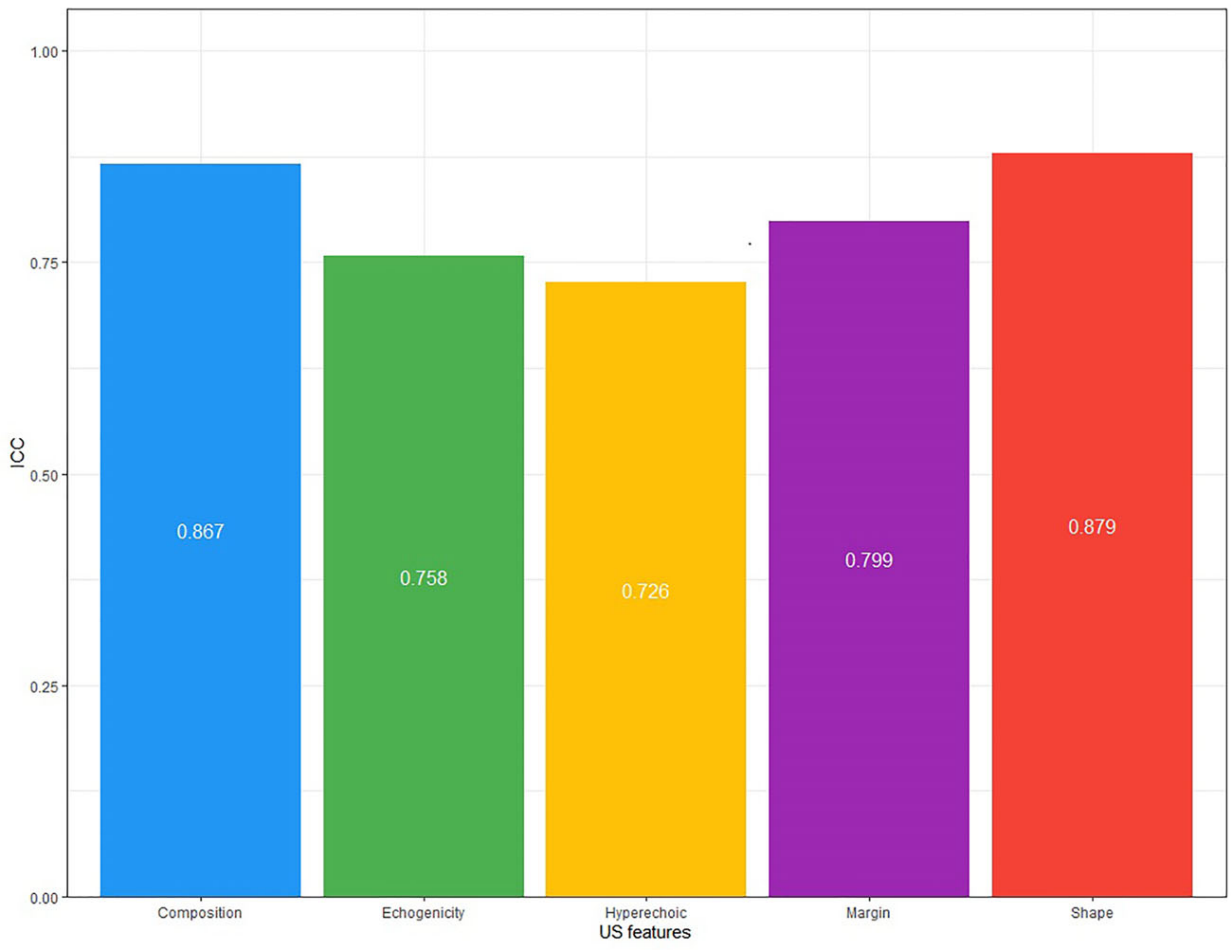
The ICC values of Ultrasound features.
